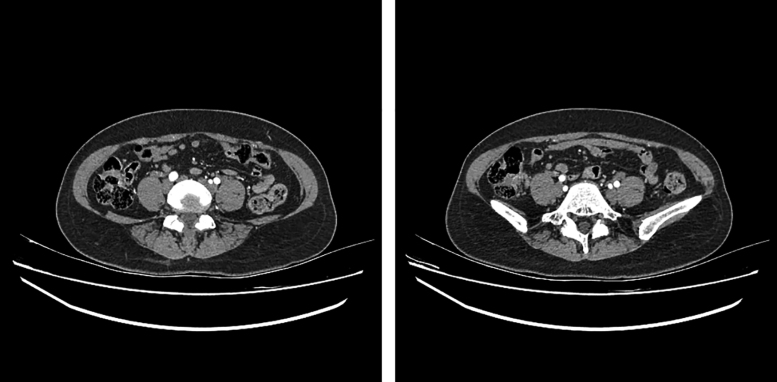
Abdominal CT scan. CT = computed tomography.
